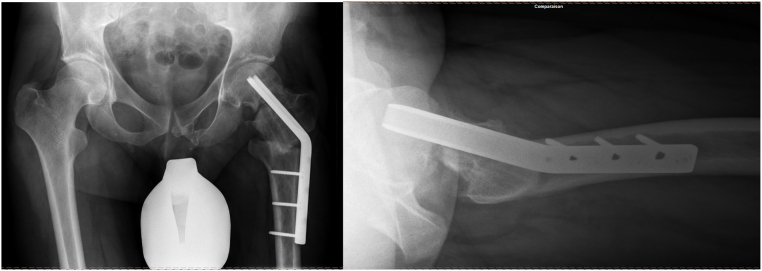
AP pelvis and cross-table lateral hip radiographs after valgus intertrochanteric osteotomy using a 130° blade plate.
